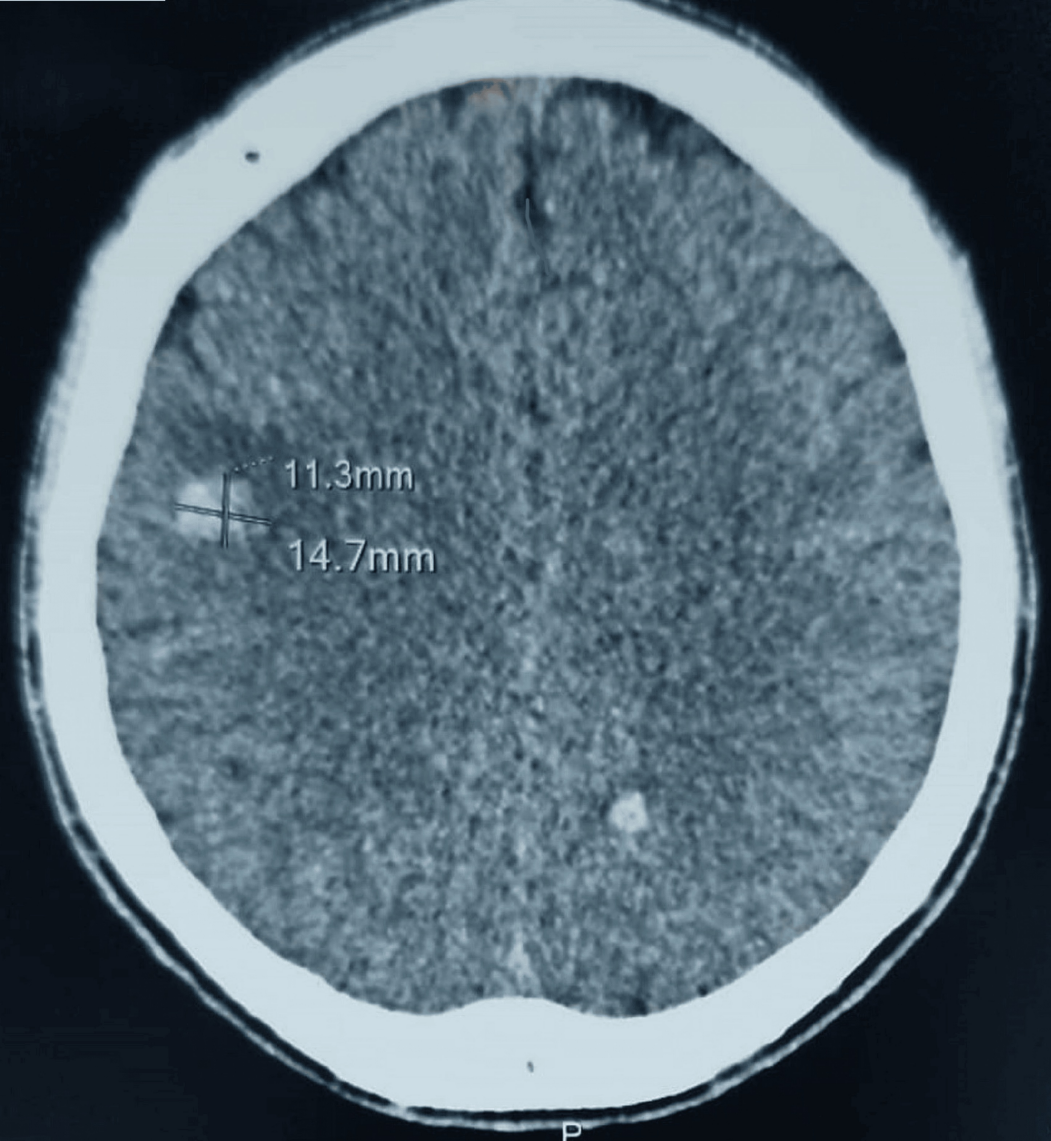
Brain CT scan Brain CT scan showing spontaneous hyperdensity in the right frontal and left parietal lobes without mass effect, suggestive of a multiple cerebral hematoma.
